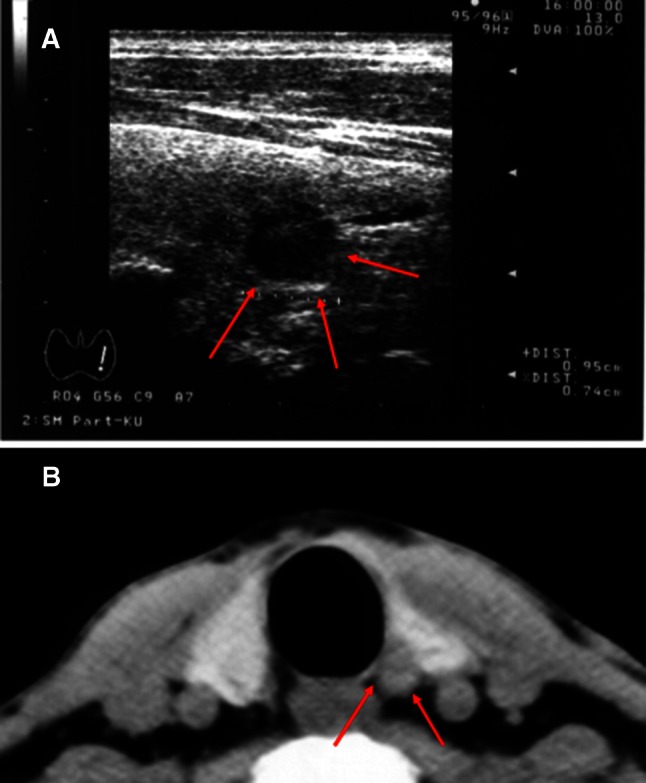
a Sonogram of a 74-year-old man with left vocal cord paralysis revealing a small tumor extending from the dorsal surface of his left thyroid lobe. b CT scan showing a small tumor located on the course of the left recurrent laryngeal nerve
